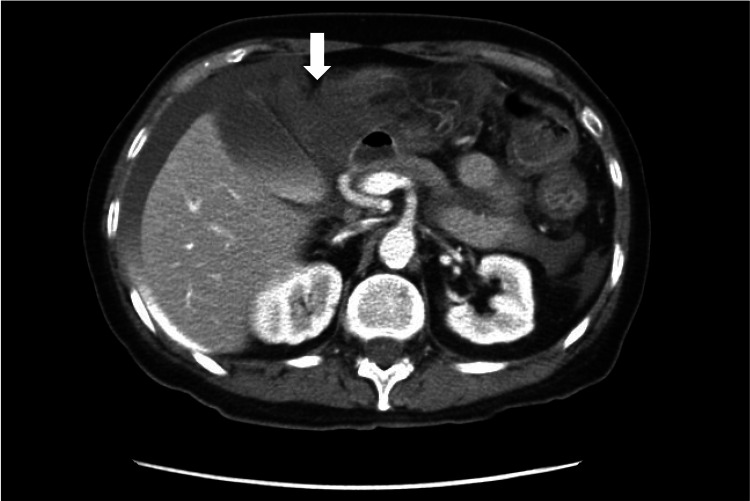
Axial contrast-enhanced CT of the abdomen demonstrating active contrast extravasation consistent with hemoperitoneum (arrow).
